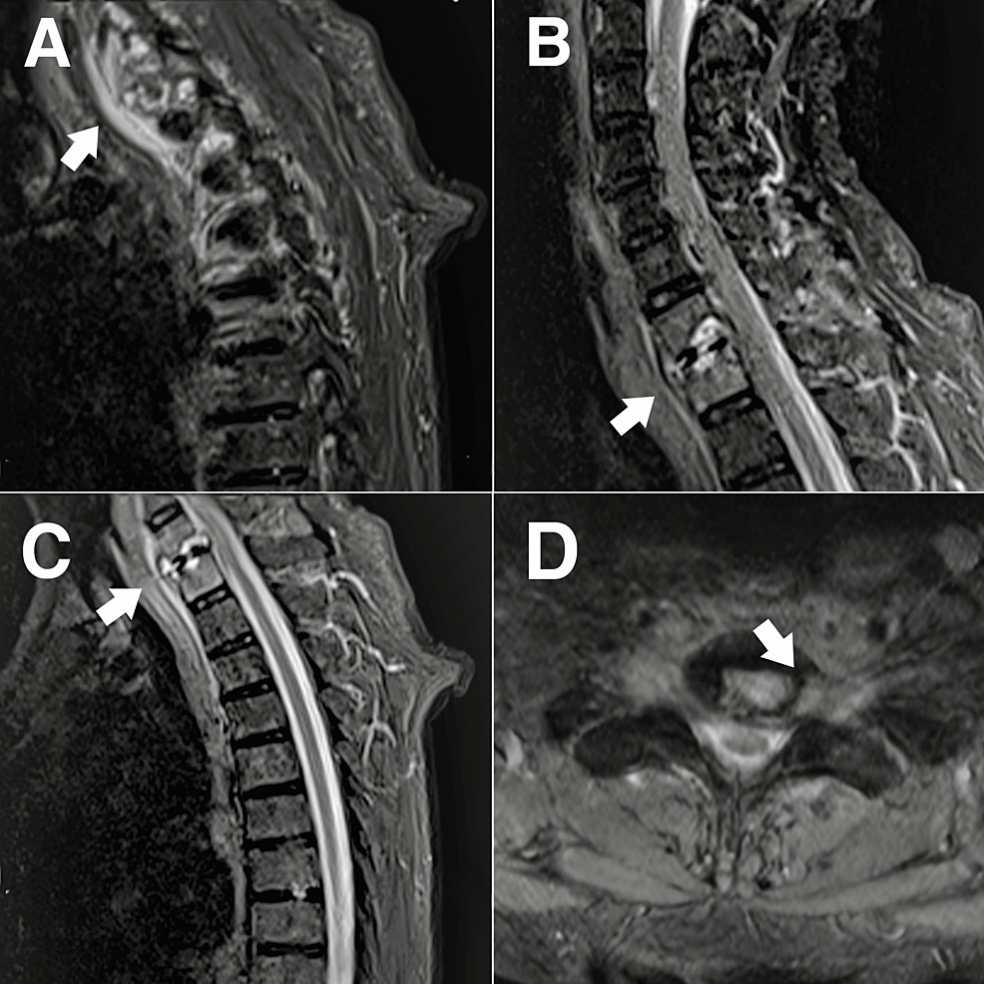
Sagittal T2-weighted magnetic resonance image (MRI) (A) Increased paravertebral soft tissue thickening/fluid collection is noted from C6/7 to T3/4 levels. (B) Increased T2-weighted hyperintensity at C7/T1 intervertebral disc with disc height loss and irregular C7/T1 endplate at the cervical scan. (C) Increased T2-weighted hyperintense signal at C7 and T1 vertebral bodies at the thoracic scan. (D) Increased paravertebral fluid collection at the C7/T1 level in the coronal view.
